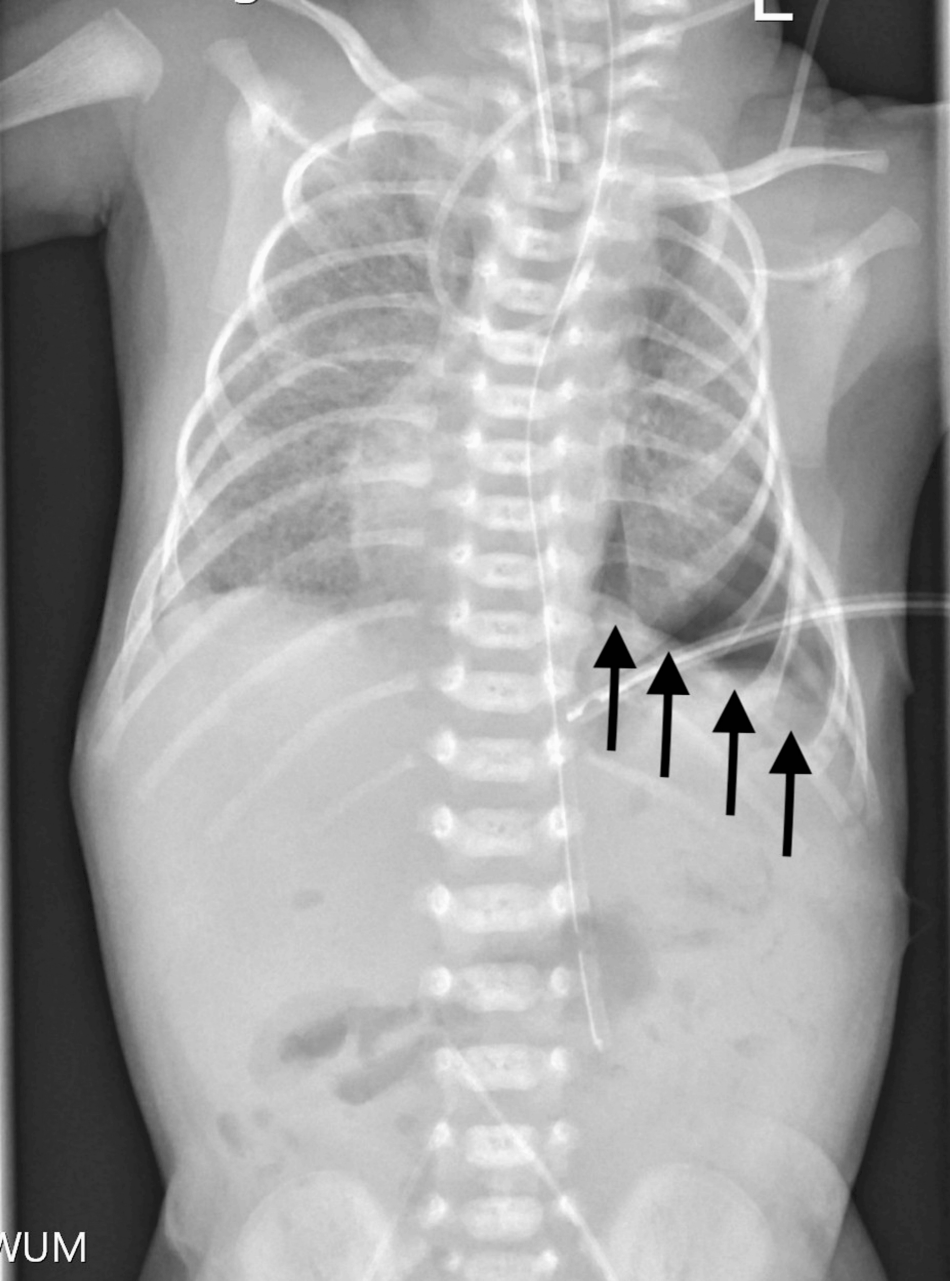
The X-ray after diaphragmatic reconstruction The arrows point to the reconstructed diaphragm.
